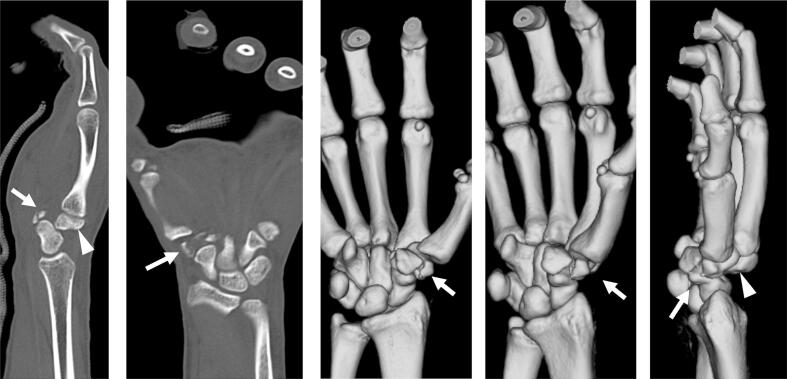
Computed tomography scan demonstrates trapezium fracture (white arrows) and dislocation of the trapezoid (white arrowheads).
